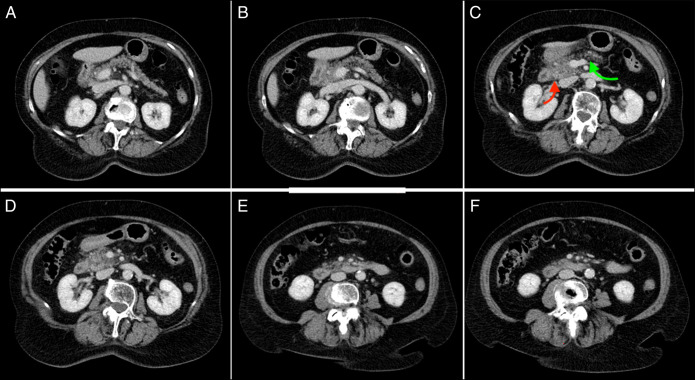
(A–F) Computed tomography abdomen findings. Red arrow denotes inflamed pancreas, and green arrow denotes normal pancreas.
